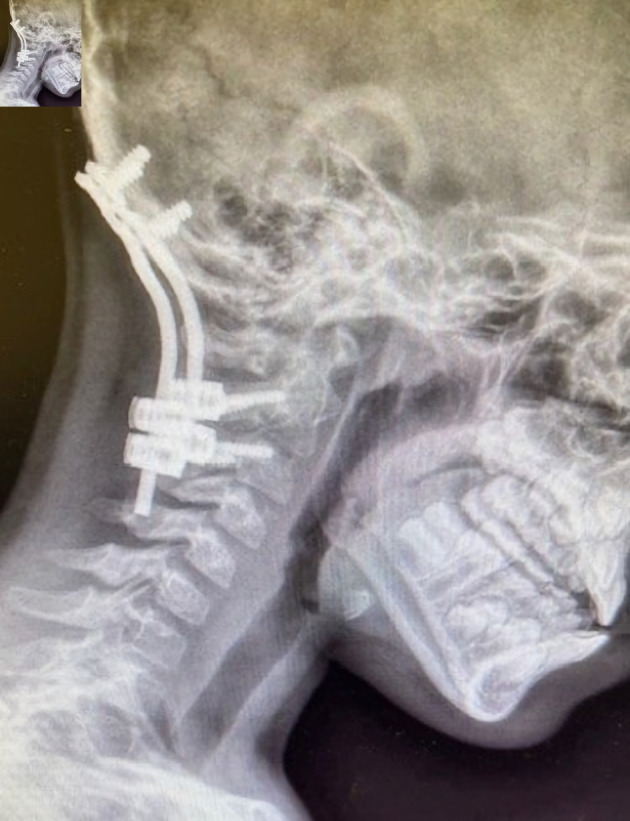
Preoperative radiographs demonstrating cervical spine instrumentation for stabilization following atlanto-occipital dislocation.
